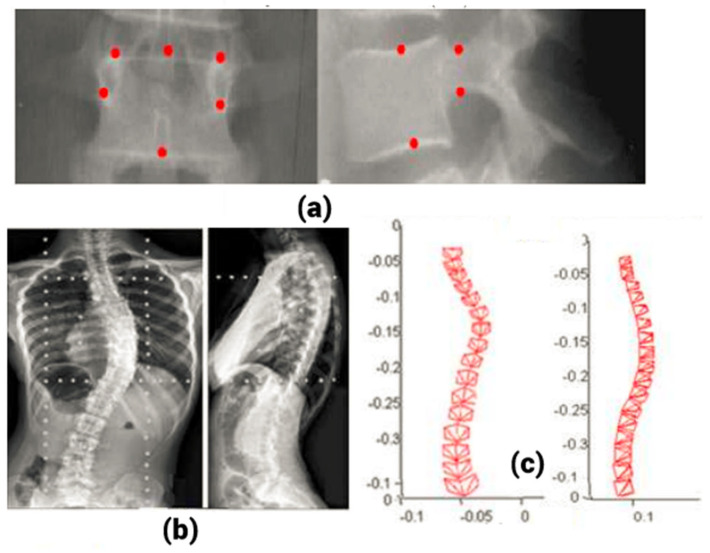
(a) Stereo-corresponding points on the vertebra used for SCP reconstruction, (b) sample biplanar radiographs, (c) SCP reconstruction [28]. SCP stereo corresponding point.
